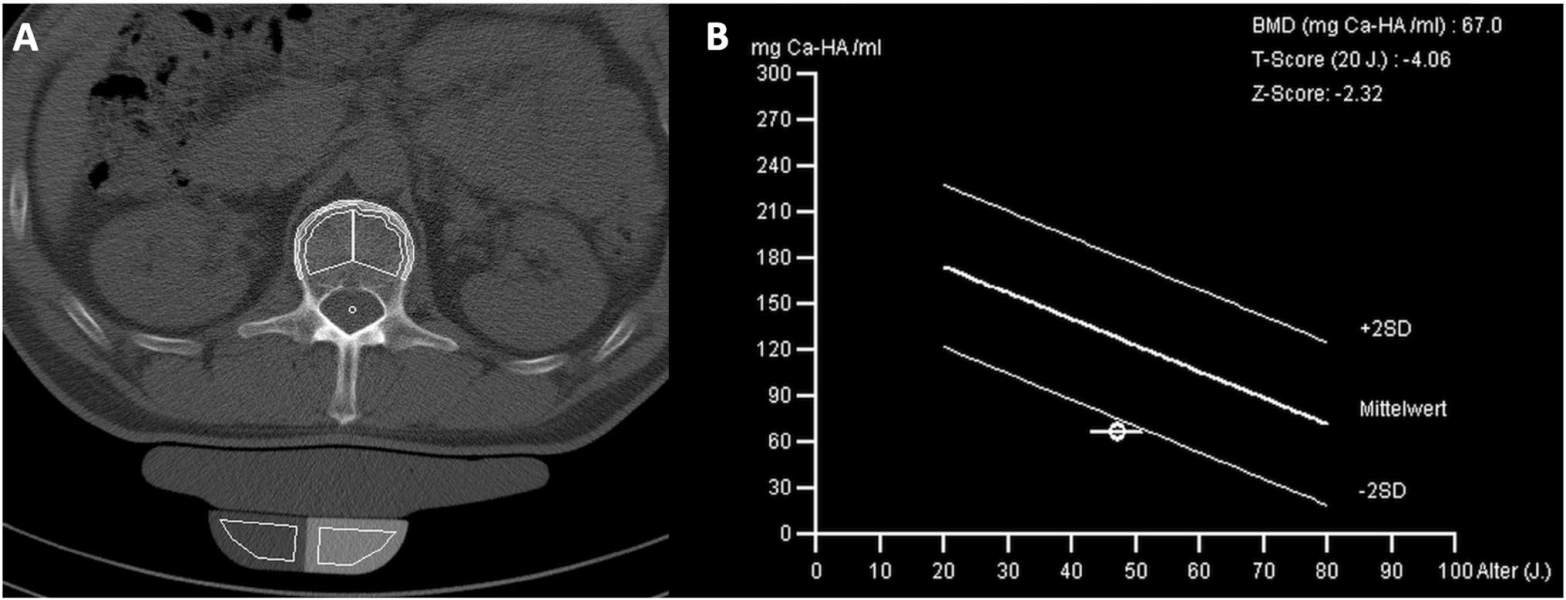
a Computed tomography scan of L1 and b bone mineral density (BMD) in a 56-year-old male patient with indolent systemic mastocytosis (ISM) and severe osteoporosis according to the traditional WHO criteria (*T* score: − 4.06). Also, a mastocytosis-related low BMD is present with a *Z* score of − 2.32
